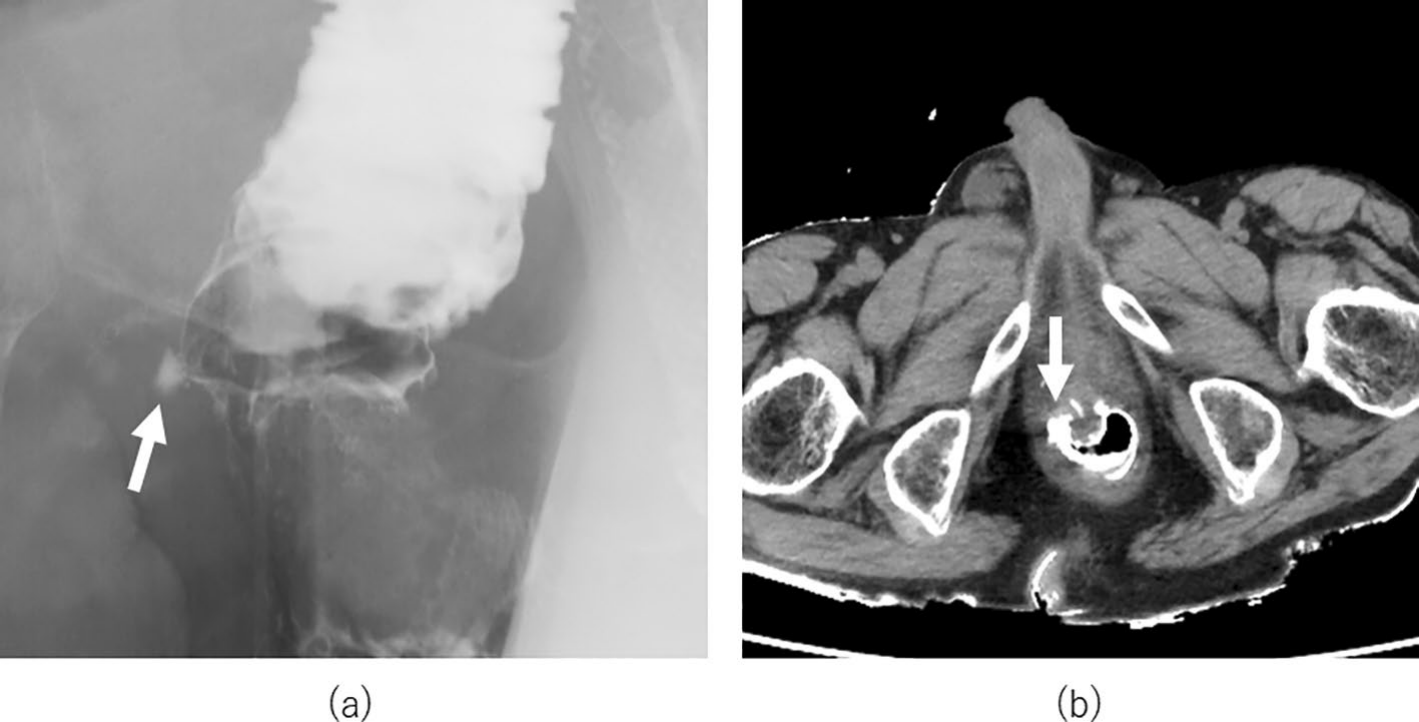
Contrast enema examination and computed tomography. A case of suspected anastomotic leakage is demonstrated. (a) The contrast medium flowed out of the intestinal tract. (b) The dehiscence of the anastomosis is difficult to identify on computed tomography.
